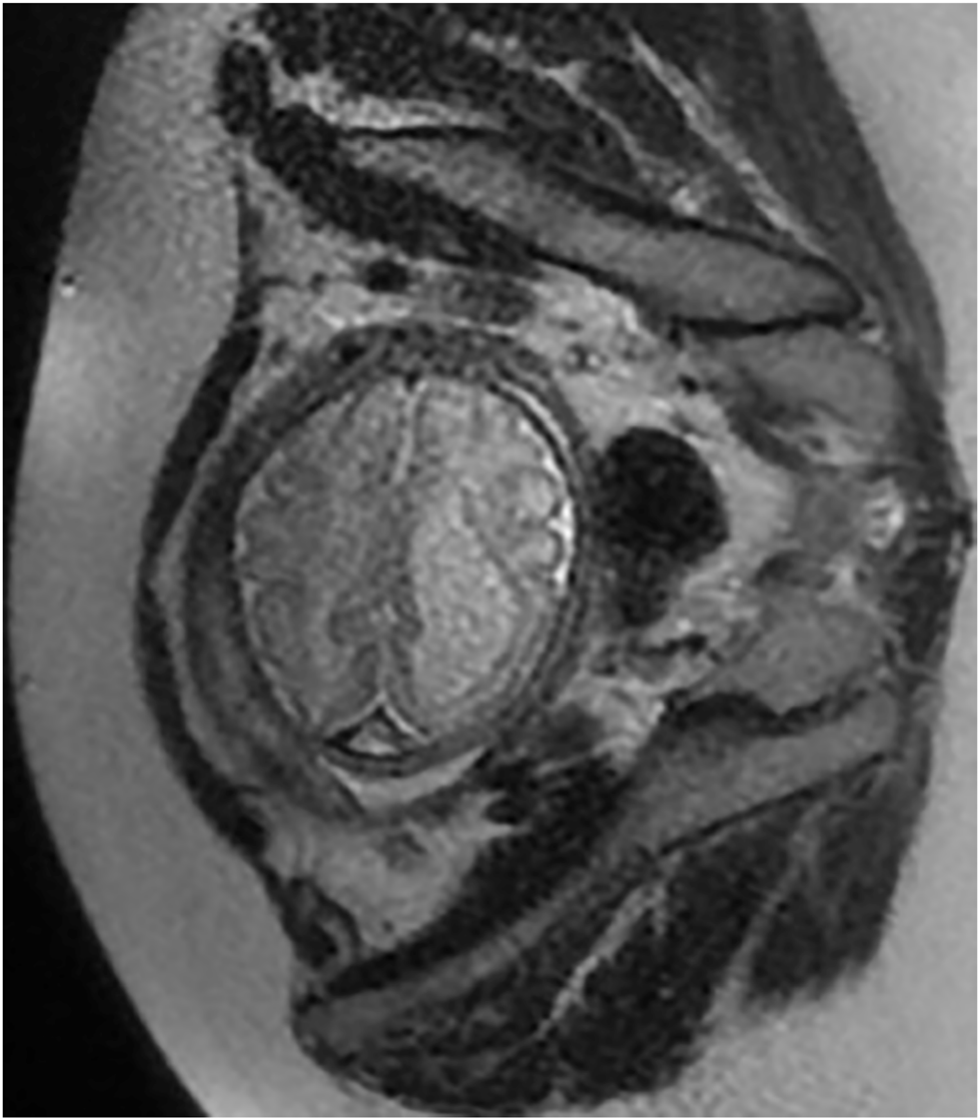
Fetal MRI at week 32 showing axial view of asymmetric ventriculomegaly.
